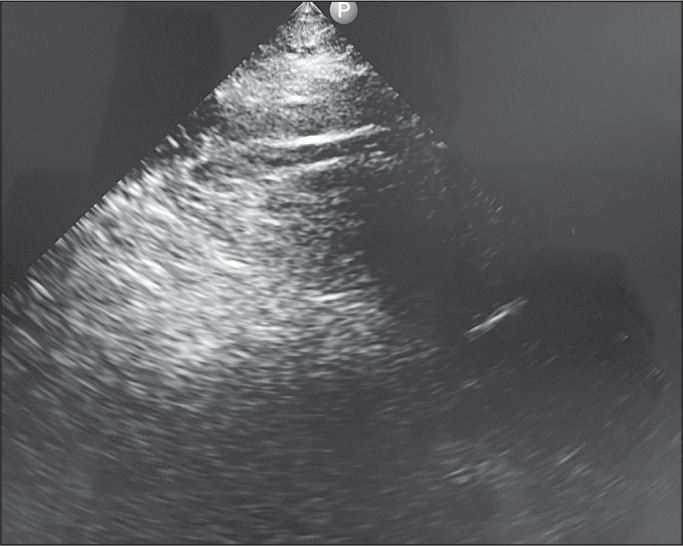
Set of three screenshots considered adequate for remote interpretation. A: Parasternal long-axis view of the heart. B: Single-quadrant view of a lung. C: IJV.
